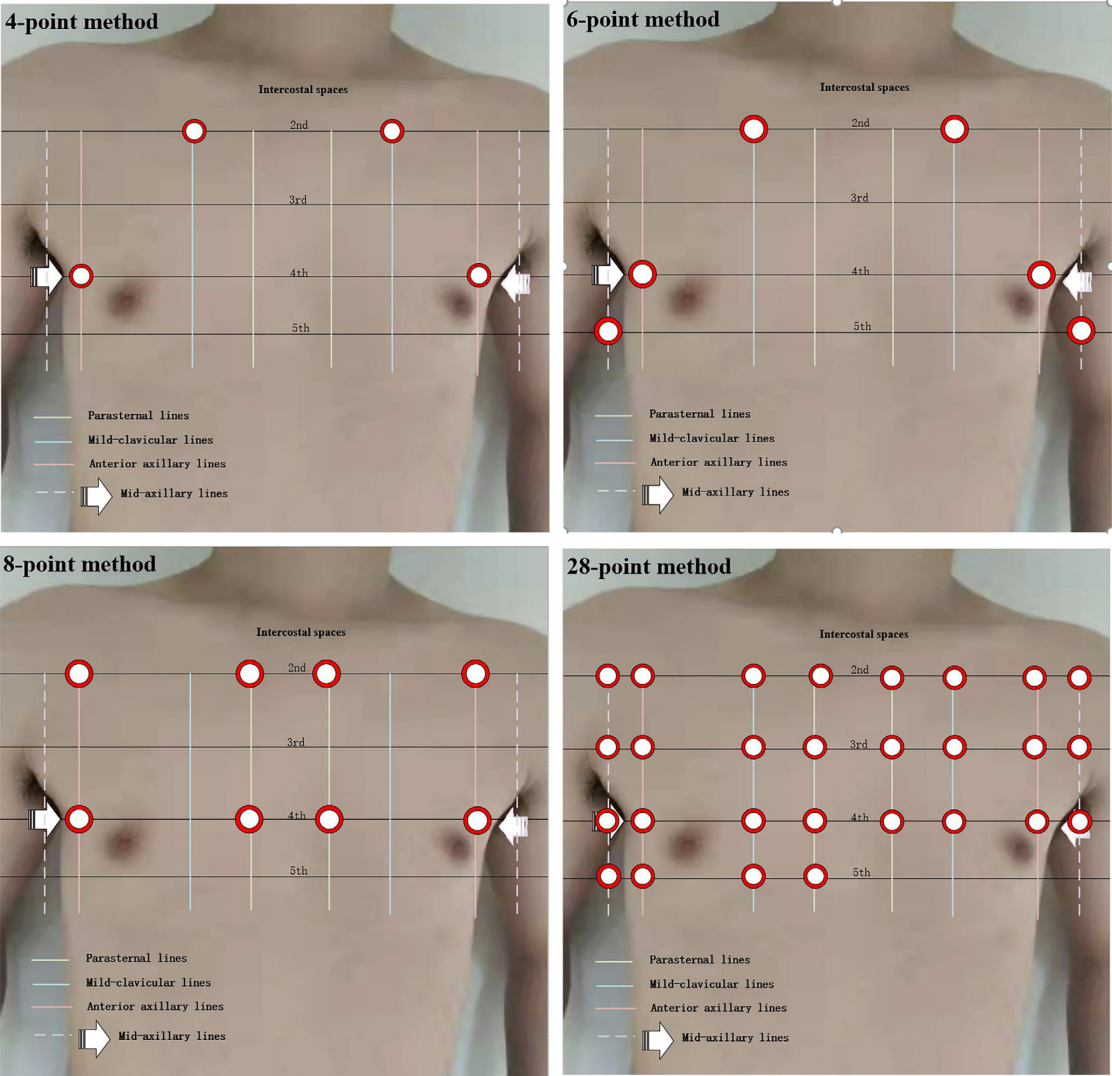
LUS methods of 4‐, 6‐, 8‐, and 28‐point method for the detection of pulmonary congestion by lung ultrasound
